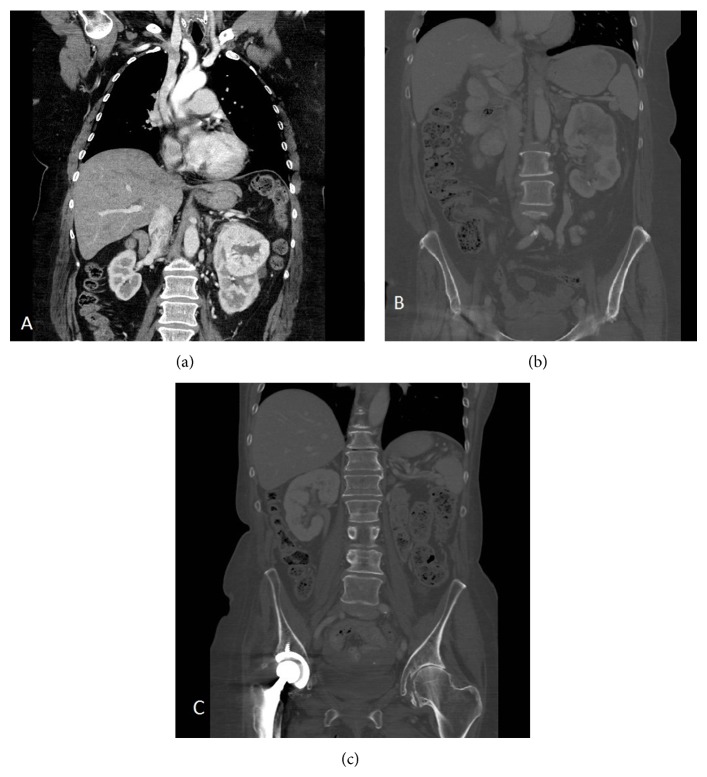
(a) Enhanced CT finding on diagnosis demonstrating the large tumour arising from the upper pole of the left kidney. (b) CT abdomen and pelvis after TKI administration: note the shrinking of the primary tumour. (c) Latest CT abdomen, as part of postoperative follow-up.
